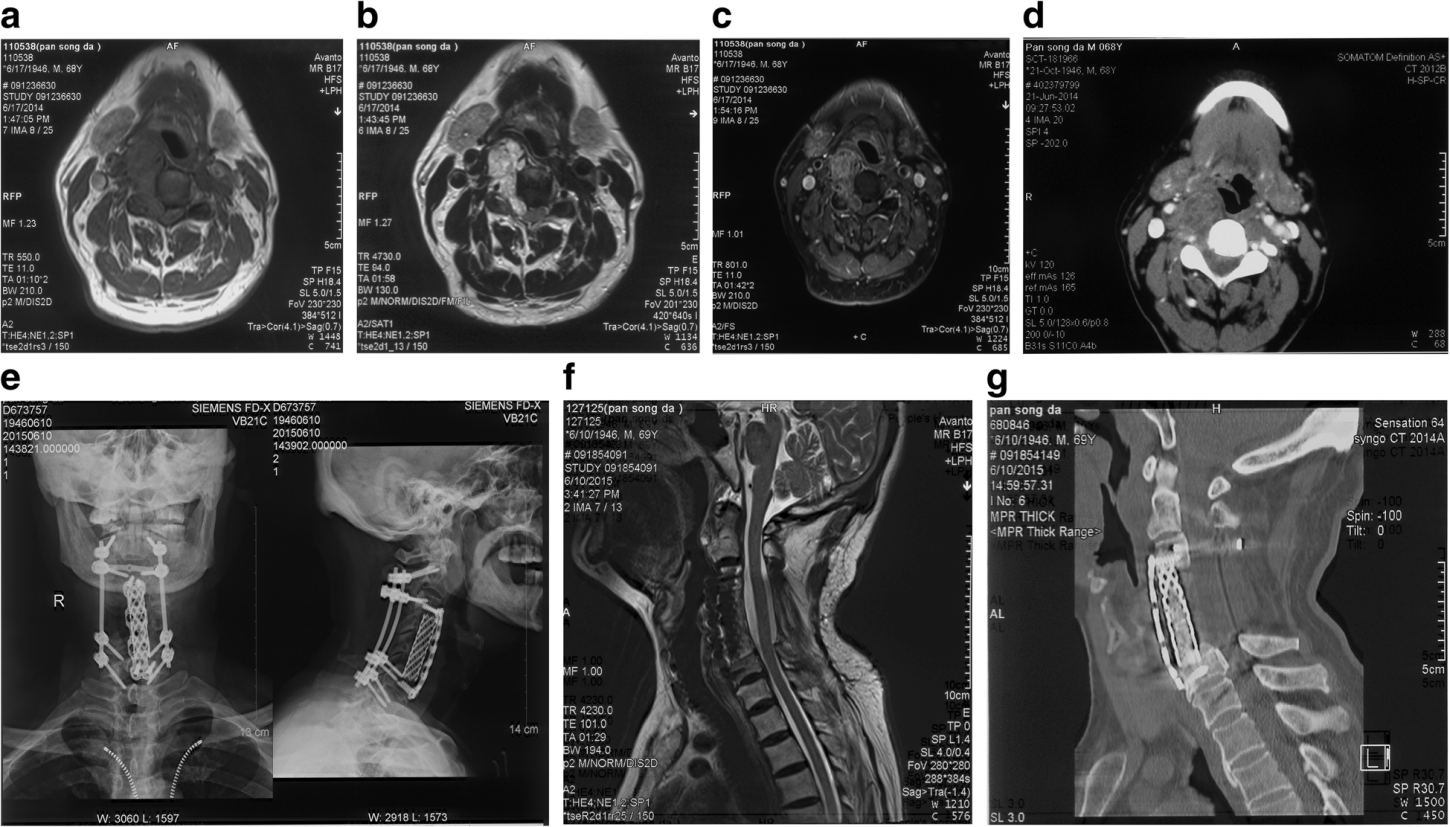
The preoperative and postoperative images. a, b A dumbbell-shaped and capsuled tumor was showed on T1 and T2, respectively. c Slight enhancement after injection of gadolinium. d Very little secondary involvement of the bone. e, f, g Postoperative X-ray, MRI, and CT
